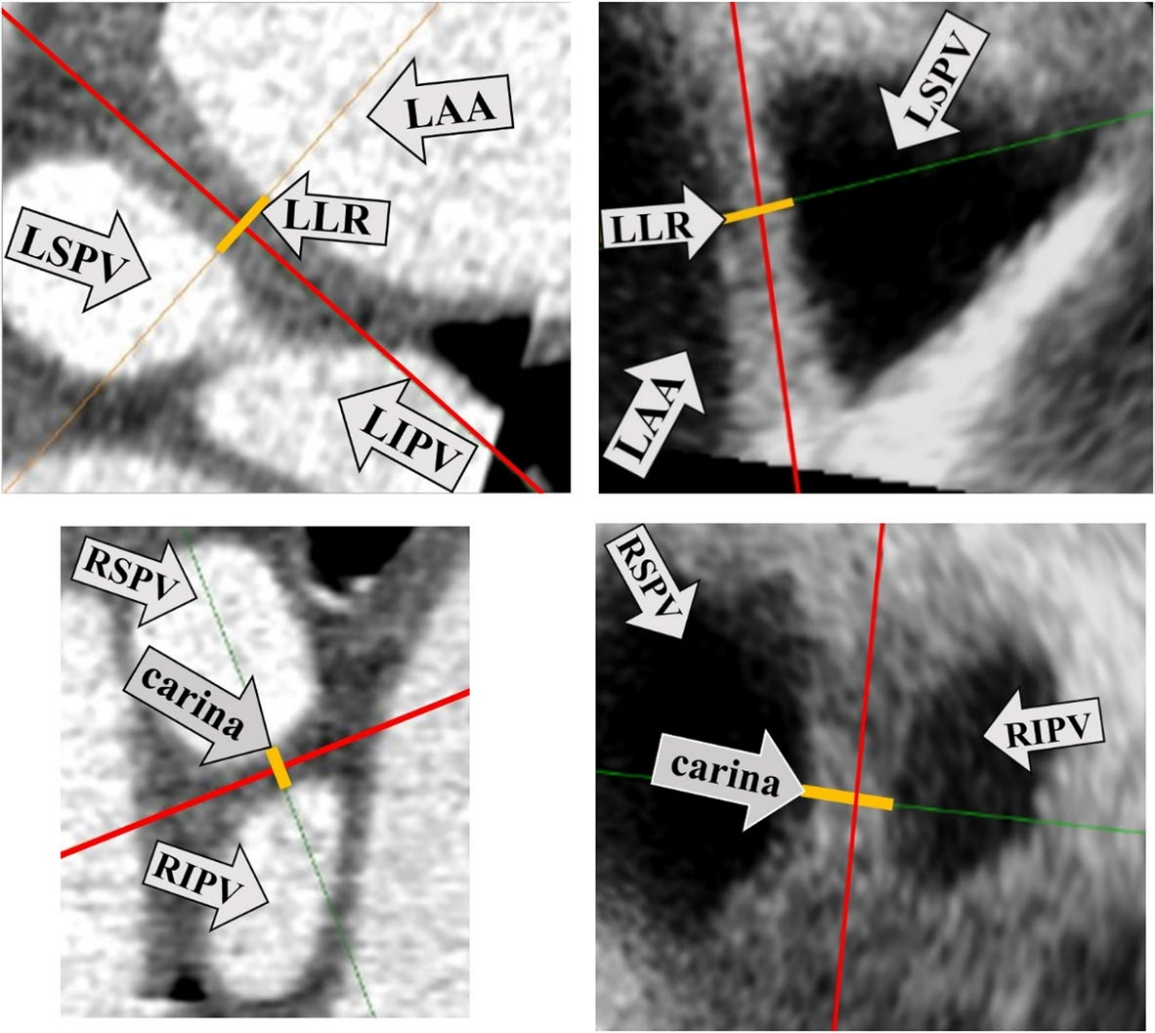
Measurement of the shortest distance (yellow line) perpendicular to the longitudinal axis (red line) of the LLR and the carina. Upper panel: Measurement of LLR width between the LSPV and the LAA from CCT (3a left) and from 3DTOE (3b right) images. Lower panel: Measurement of carina width between the RSPV and the RIPV from CCT (3c left) and from 3DTOE (3d right) images. *LLR* (Left lateral ridge), *LSPV* (Left superior PV), *LIPV* (Left inferior PV), *LAA* (Left atrial appendage), *CCT* (Cardiac computed tomography), *3DTOE* (Three-dimensional transesophageal echocardiography), *RSPV* (Right superior PV), *RIPV* (Right inferior PV)
